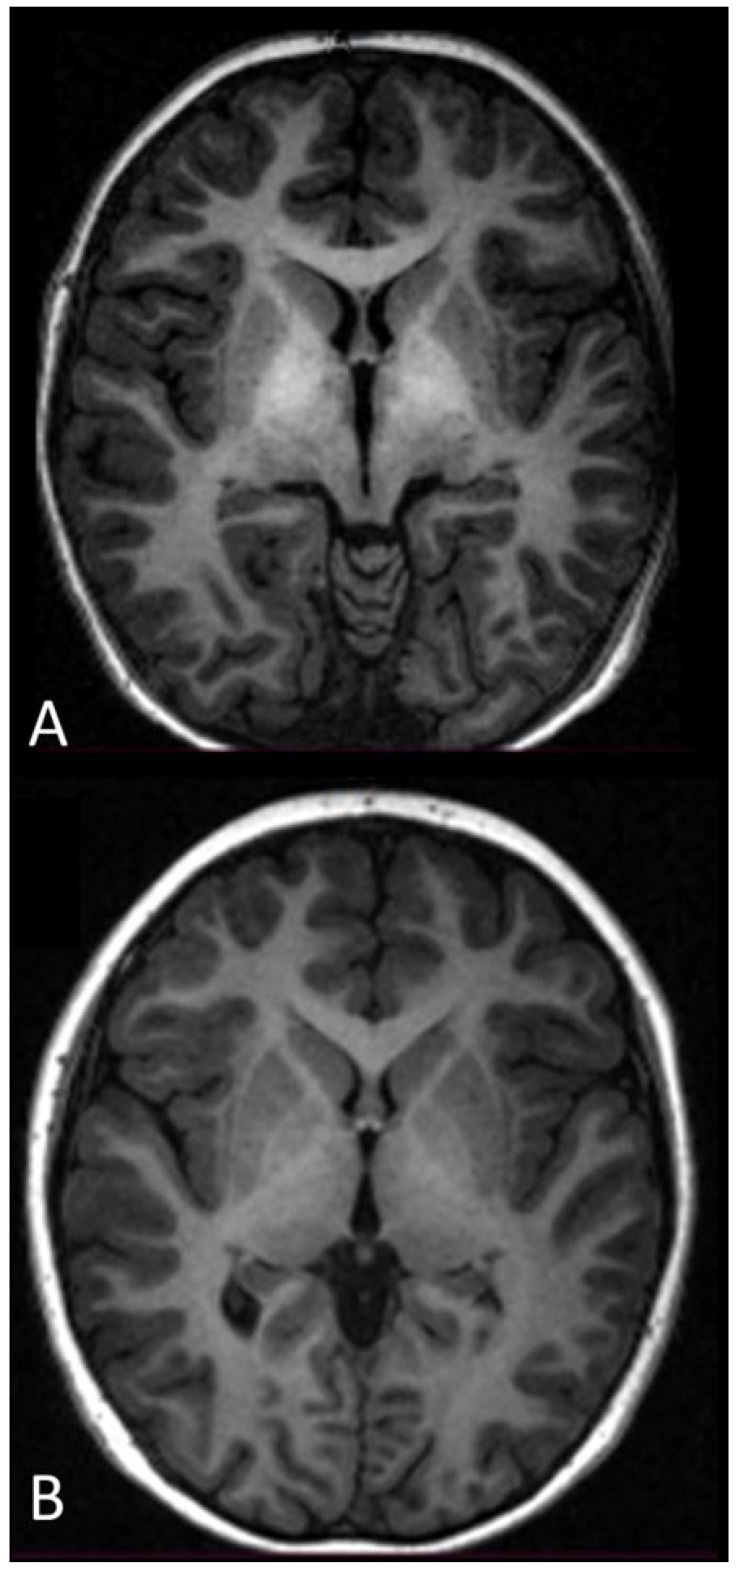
Axial T1-weighted MR image at the level of the basal ganglia of the brain (A) before surgery and (B) after surgery. (A) Bilateral symmetric hyperintensity in the globus pallidus on the T1-weighted MR image was observed in all cases in this series. (B) Bilateral symmetric hyperintensity in the globus pallidus on the T1-weighted MR image disappeared in the two patients who had follow-up >12 months.
